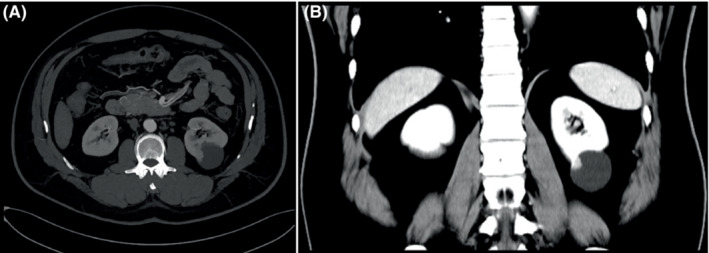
Computed tomography of succinate dehydrogenase‐deficient renal cell carcinoma. (A) Plain computed tomography showed that the cystic‐solid mass was low density and the solid component of the mass was approximately 37 HU. (B) Enhanced computed tomography showed that the solid component of the mass was obviously enhanced with 99 HU.
